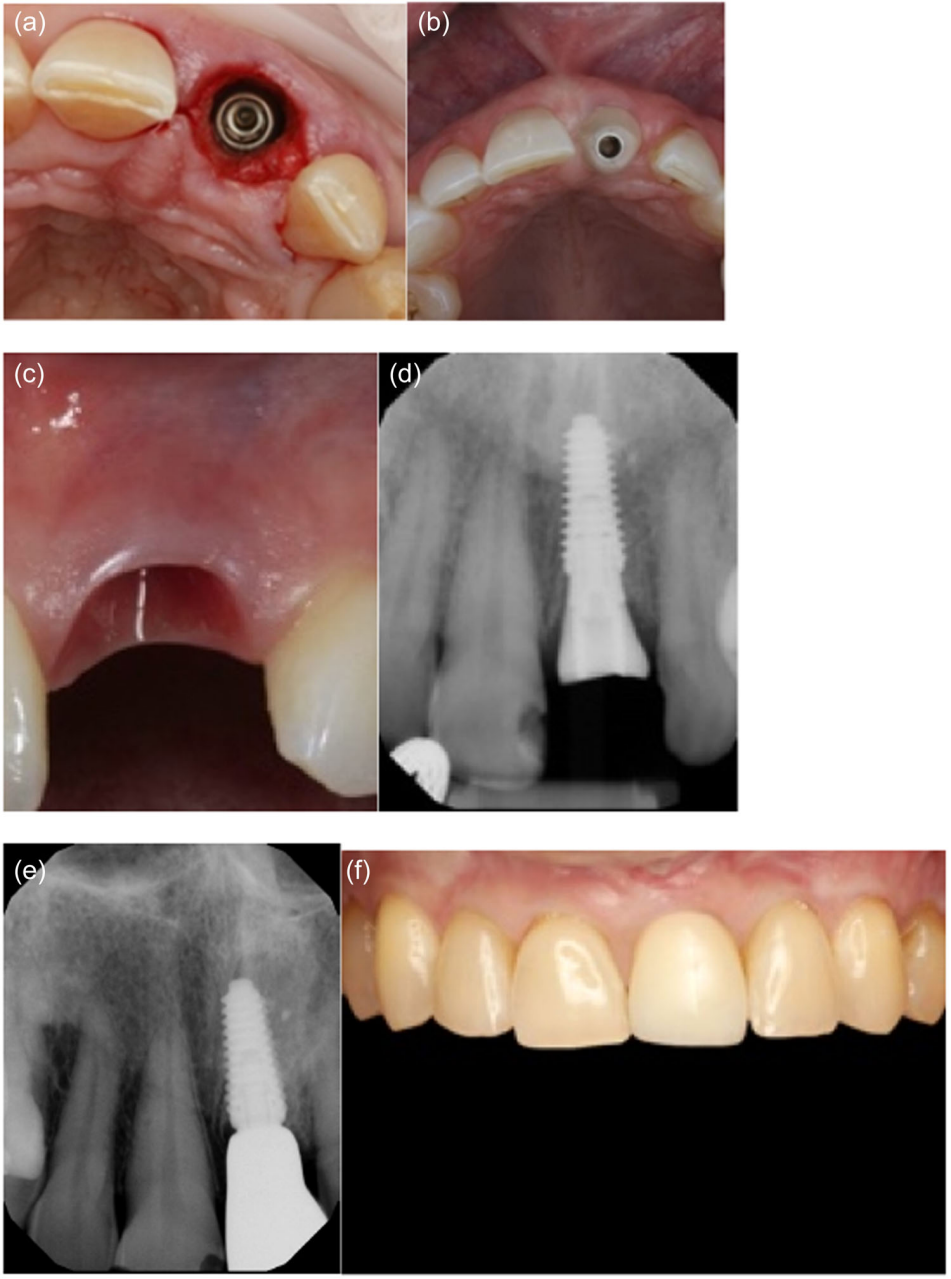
Minimally invasive removal of fractured central incisor and placement of immediate implant without osteotomy on Patient 1: (a) Immediate prepless immediate implant on central incisor. (b) Anatomical healing abutment placed. (c) Transmucosal tunnel and emergence profile development by anatomical healing abutment. (d) Peri‐apical radiograph on the day of implant placement with anatomic healing abutment. (e) Peri‐apical radiograph with final restoration at 12 months. (f) Definitive monolithic Zr restoration.
